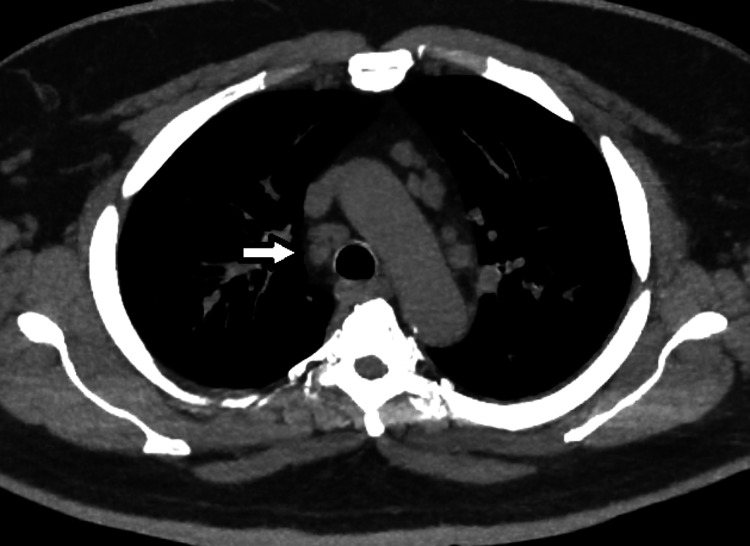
CT chest (mediastinal window) above the carina. Mediastinal lymphadenopathy (arrow).
